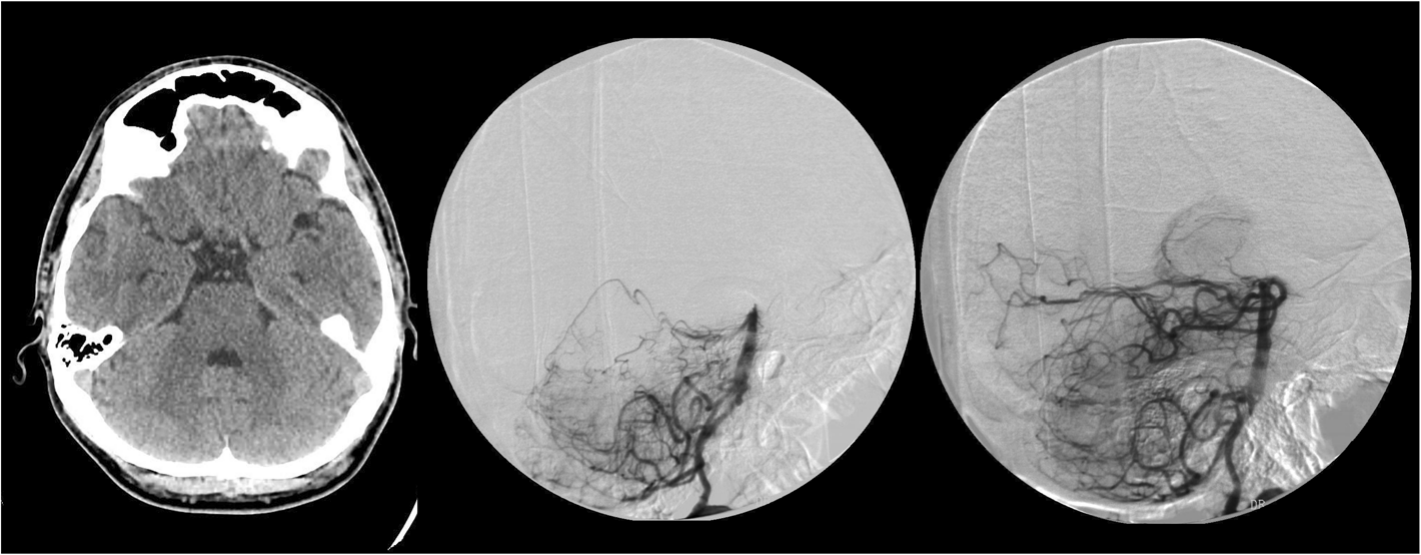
Case 1: Non-enhanced cranial CT showed no acute ischemic signs or hemorrhage. Digital subtraction angiography revealed basilar artery occlusion (A) and successful recanalisation after intra-arterial thrombolysis with 25 mg rtPA
